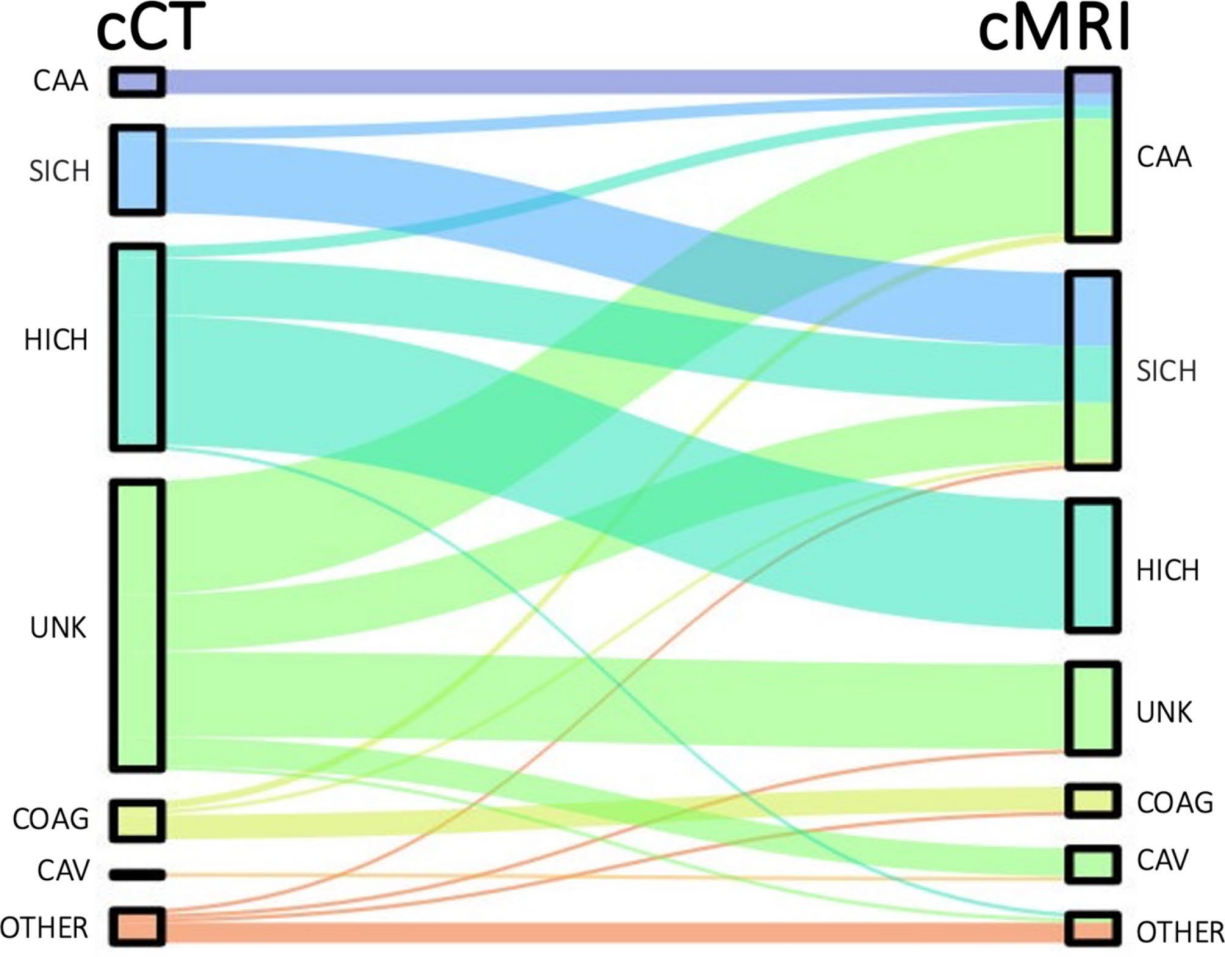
Sankey Plots visualizing the change of attributed etiology after performing an additional MRI scan. The graph was created using Microsoft PowerPoint^®^ 2022. CAA: Cerebral Amyloid Angiopathy, SICH: Secondary Hemorrhagic Transformation in Ischemic Stroke, HICH: Hypertensive ICH, UNK: Unknown, COAG: Coagulopathy, Cav: Cavernoma, Other: includes Neoplasm, Arteriovenous Malformation, Aneurysm, Vasculitis, Trauma, Sinus Vein Thrombosis, and others
